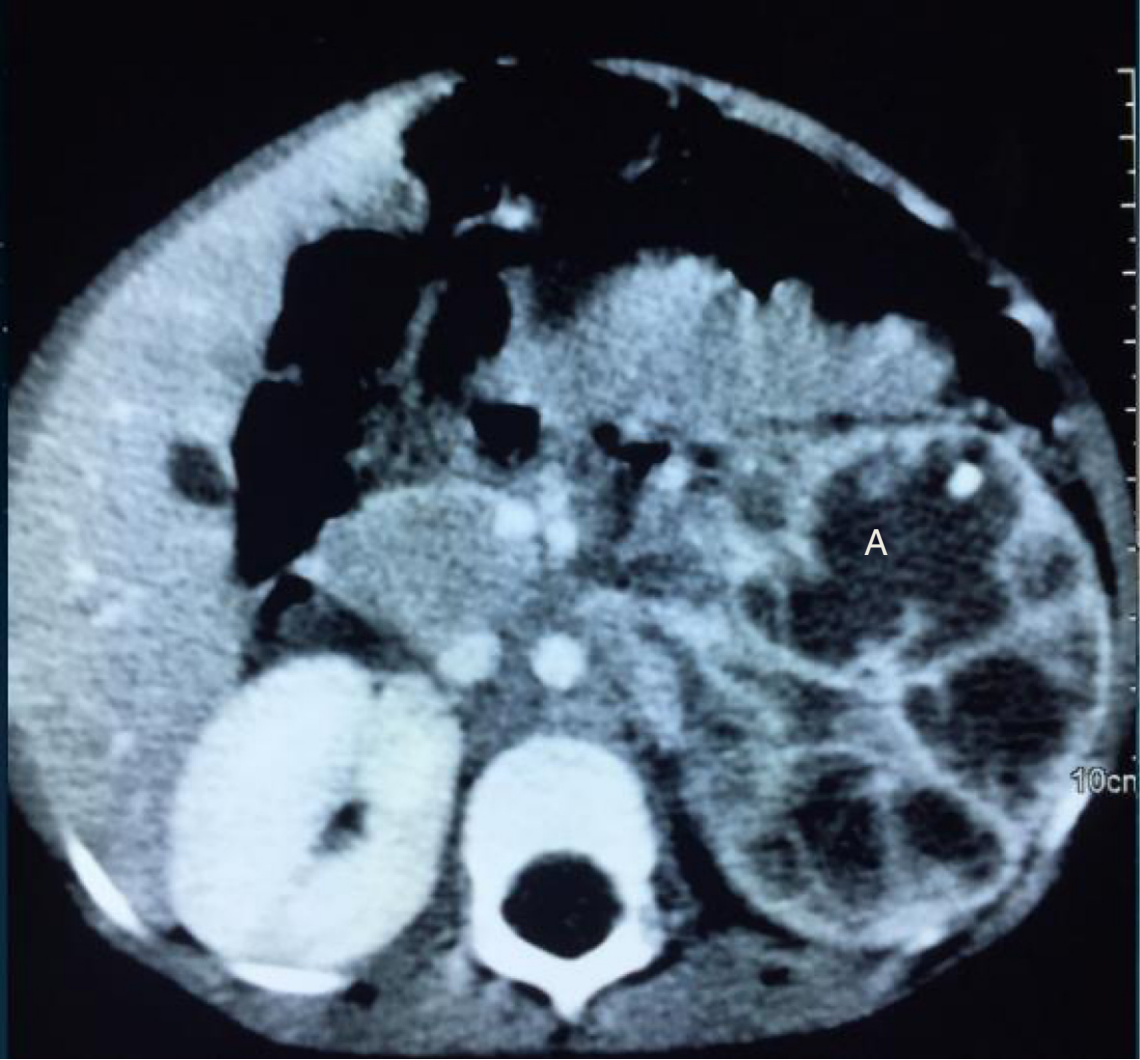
Contrast enhanced abdominal CT-scan showing multiple cysts in the left kidney (A) with peripheral enhancement of residual normal renal tissuesᅟ
